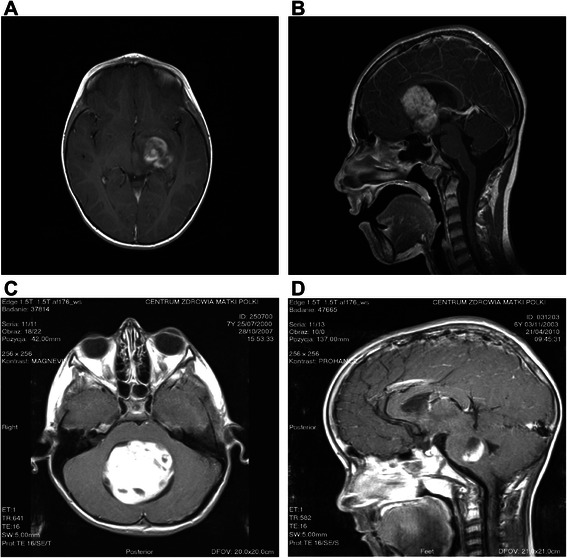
Location of pilocytic astrocytoma. a cerebral hemispheric tumor. b optic tract and hypothalamic tumor. c cerebellar tumor. d brainstem tumor. MRI scans after contrast administration
